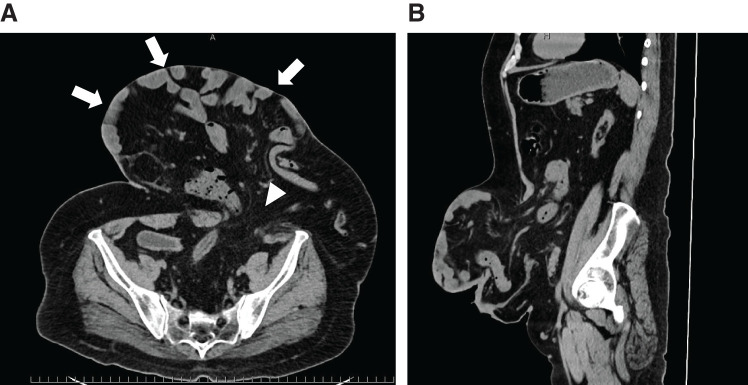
(A, B) CT findings. CT shows a giant hernia around the stoma with contents of the colon and small intestine(➡). The hernia portal is approximately 9 × 9 cm (▲).
